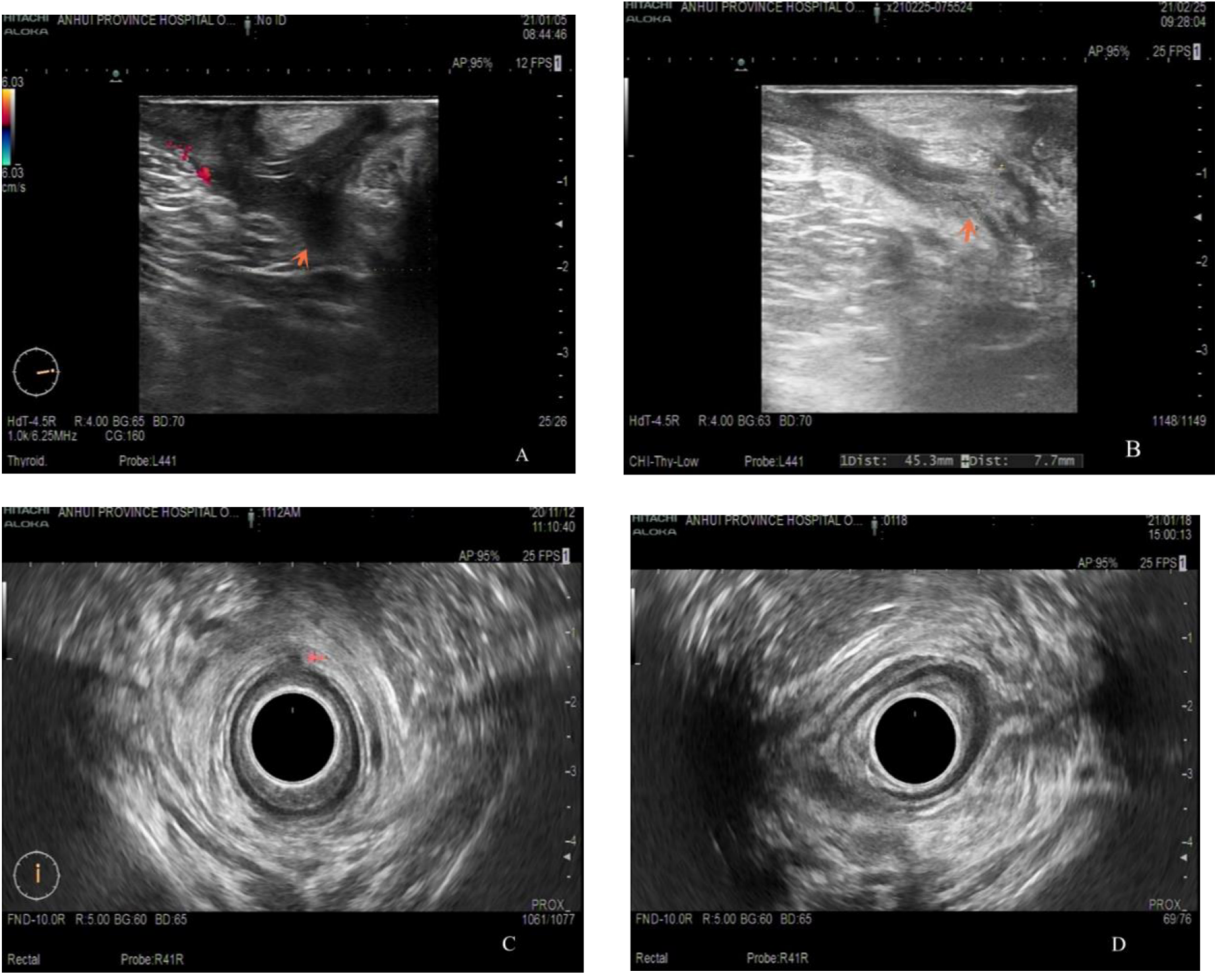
Dual-plane color ultrasound images of the anal canal of a young male before (A,C) and after (B,D) receiving NiTi-AFC internal orifice closure. The orange arrow indicates that the low-echo fistula has been replaced by a high-echo scar.
